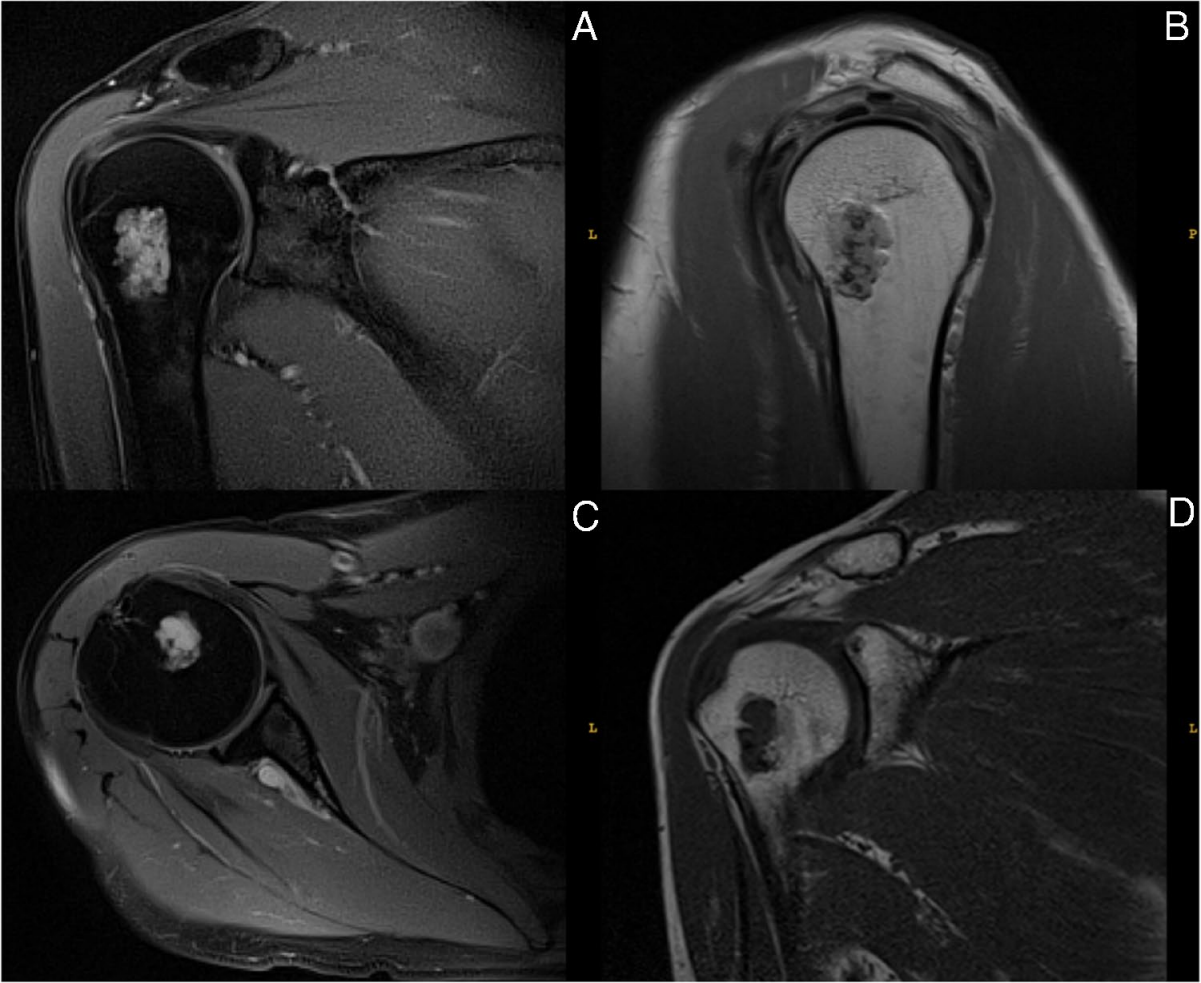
MRI scan of an enchondroma of the right humerus: A proton density, blade with fat suppression, coronal; B proton density + t2, sagittal; C proton density, blade with fat suppression, transversal; D t1, coronal, spin echo
